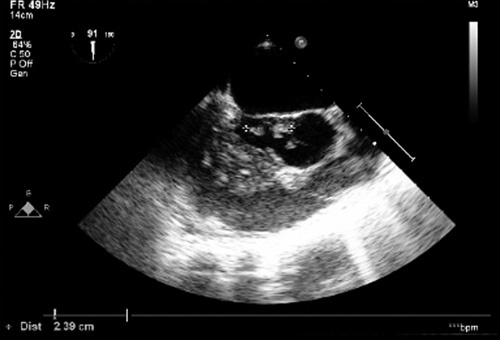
Aortic valve vegetation, seen with transthoracic echocardiogram.
